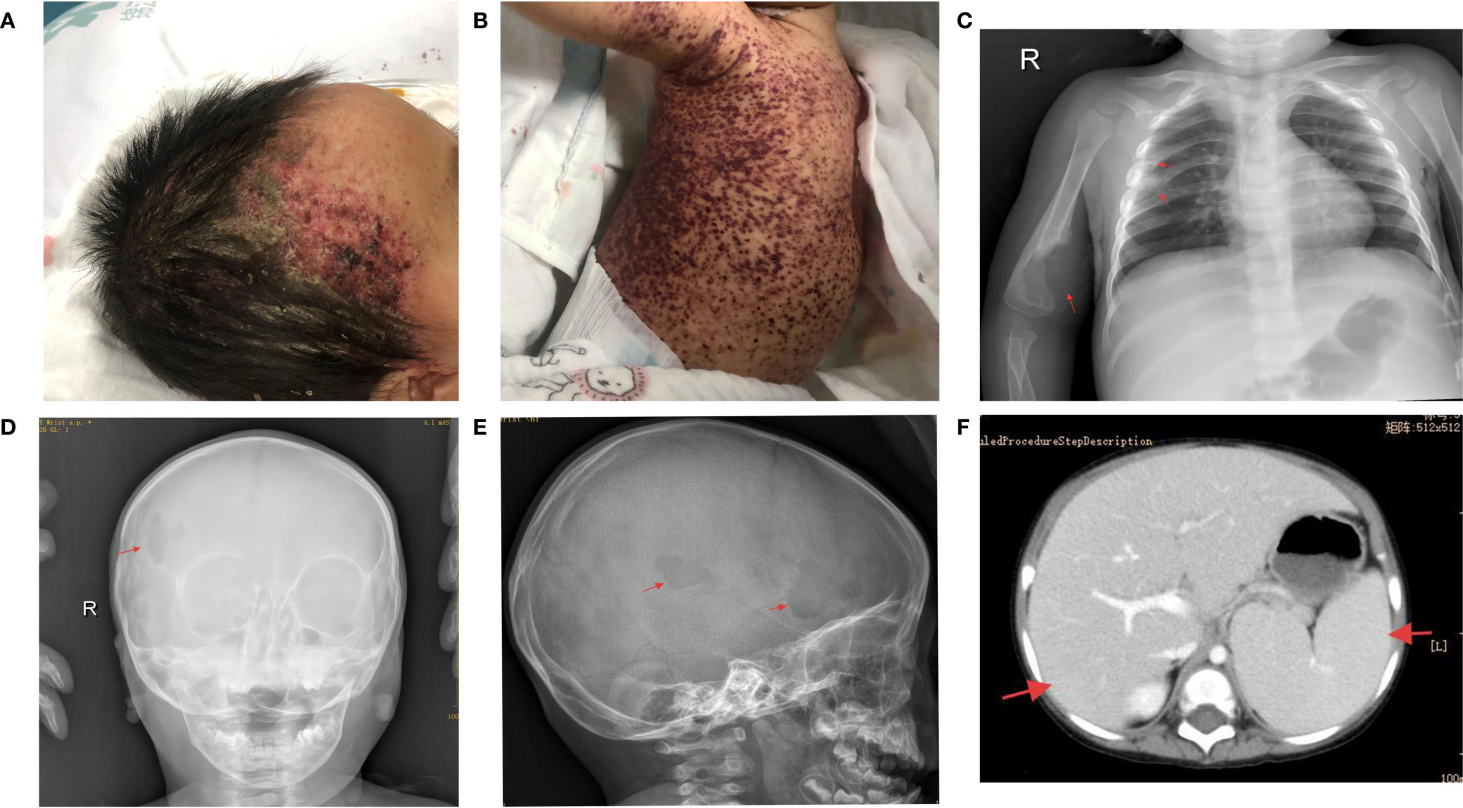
(A, B) Upon hospital admission, the patient exhibited purplish-red hemorrhagic rash and seborrheic rash on most of the trunk and head. (C) Chest X-ray showed bone destruction in the 4th and 5th ribs and right humerus. (D, E) Skull X-ray indicated bone destruction in the right frontal bone. (F) Abdominal enhanced CT revealed enlarged liver and spleen with reduced liver density.
